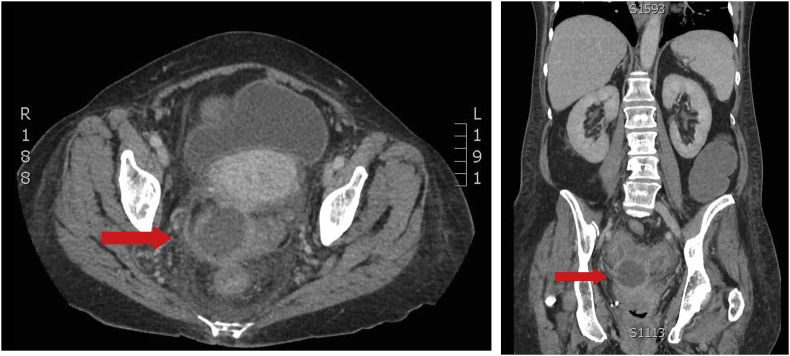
CT imaging of the abdomen revealing a 4.9cm thick-walled focal fluid collection between the posterior wall of the uterus and redundant sigmoid colon concerning for perirectal abscess.
